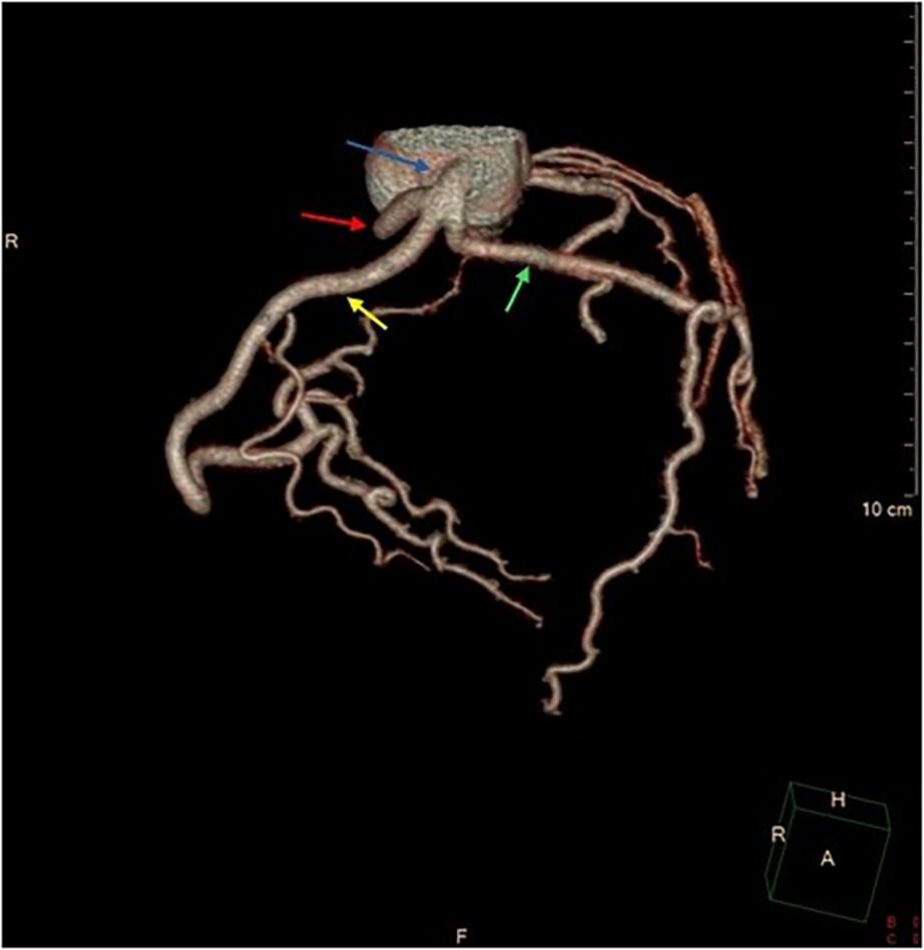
3d volume rendering cardiac CT presentation of “single” coronary artery main vessel originates from right coronary sinus (blue arrow), LAD (green arrow), LCx (red arrow) and RCA (yellow arrow).
